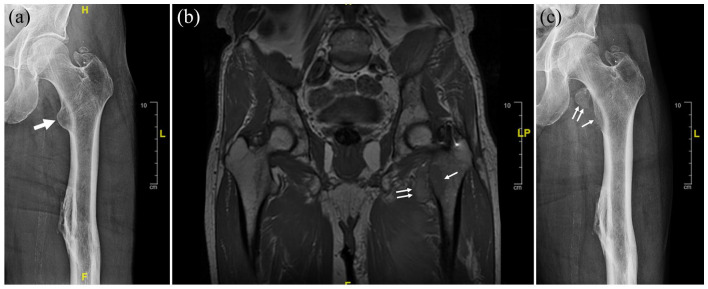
Avulsion fracture at the lesser trochanter of left femur. (a) X-ray of left hip 2 months before presentation. (b) MRI of left hip a few days after presentation showed avulsion fracture of the lesser trochanter of left femur with detachment of the iliopsoas muscle tendon from the left femur. (c) X-ray of the left hip 1 month after presentation. Thick arrow: lytic lesion on the lesser trochanter of left femur; thin arrow: remaining lytic lesion on the lesser trochanter of left femur after avulsion fracture. Double thin arrows: the detached lytic lesion from the lesser trochanter of left femur after avulsion fracture. Note the migration of the detached lesion 1 month after presentation.
